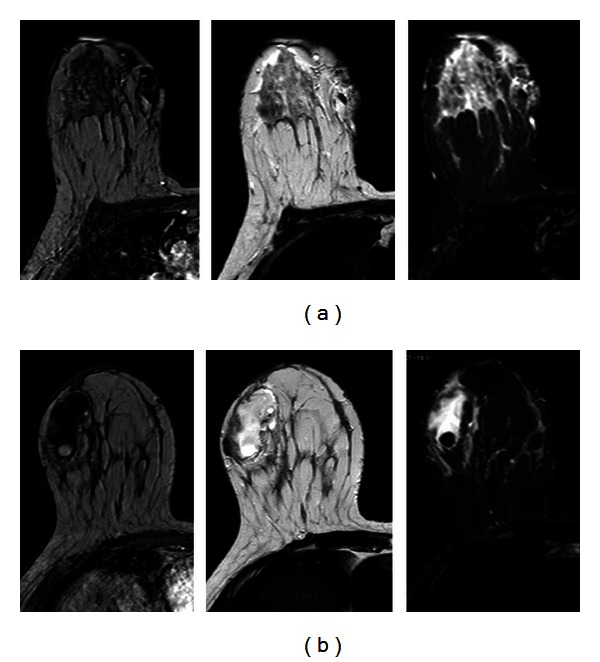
T1-weighted high resolution isotropic volume examination (THRIVE), T2-weighted sequences, and T2-weighted short tau inversion-recovery (STIR) sequences show a structural subcutaneous and cutaneous alteration with pseudonodular appearance in the upper inner quadrant of the right breast (a) and a T1 mixed hyper- and hypointense and T2 hyperintense lesion in the lower outer quadrant of the right breast, suggested for an inflammatory fluid pocket (b).
